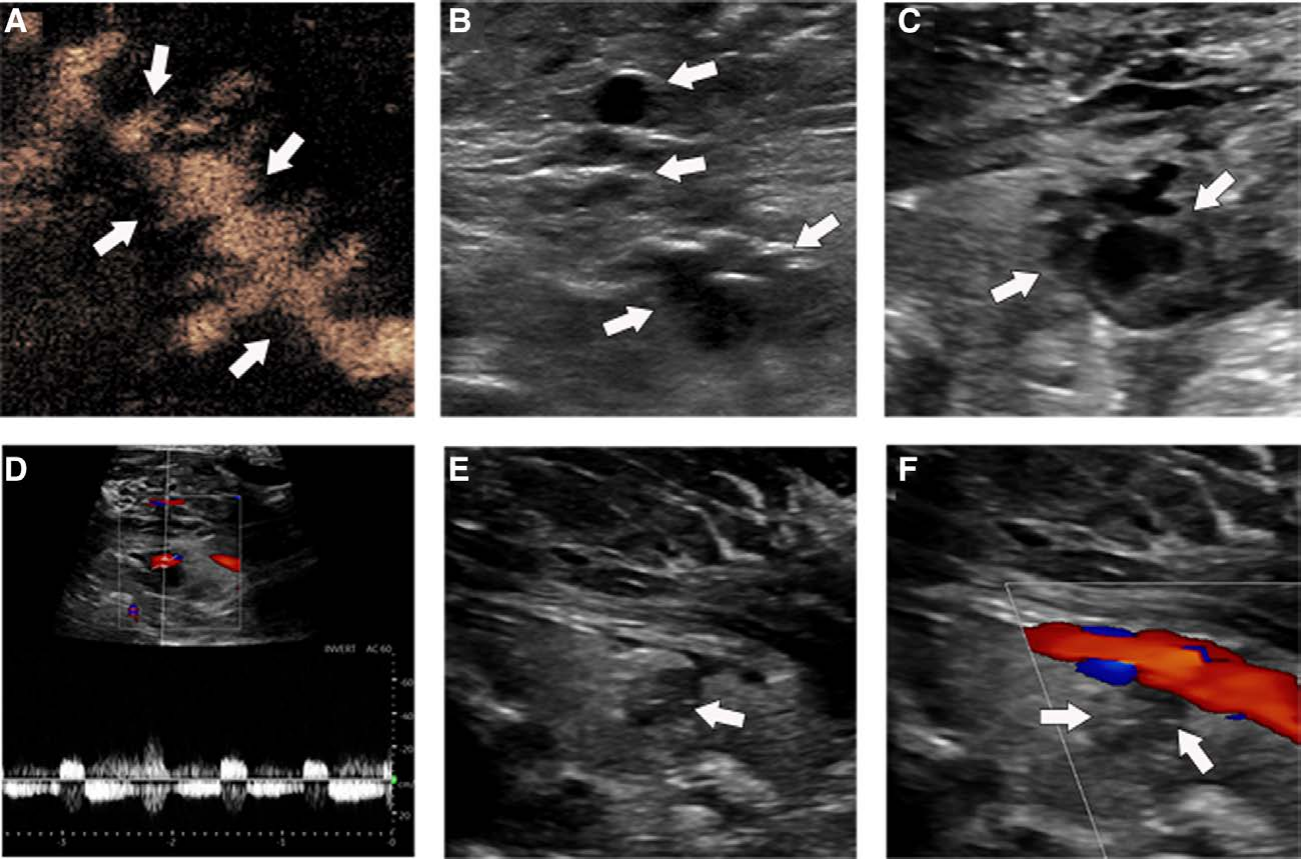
CEUS showed that Acute hemorrhage of left neck, the source of bleeding is the inferior thyroid artery (Panel A, white arrow). We can see more anechoic areas (Panel B, white arrow) on the outside of the thyroid gland, 24 hours later, a pseudoaneurysm from the inferior thyroid artery, with a size of, and thrombosis on the capsule wall (Panel C). Spectral Doppler revealed a typical to-and-fro flow (bidirectional) (Panel D). 72 hours later, US showed that a thrombus filled the whole cavity, and smaller than before (Panel E, white arrow) CDFI showed that no blood flow entered the tumor (Panel F, white arrow). CDFI = Color Doppler imaging, CEUS = contrast-enhanced ultrasound, US = ultrasonography.
